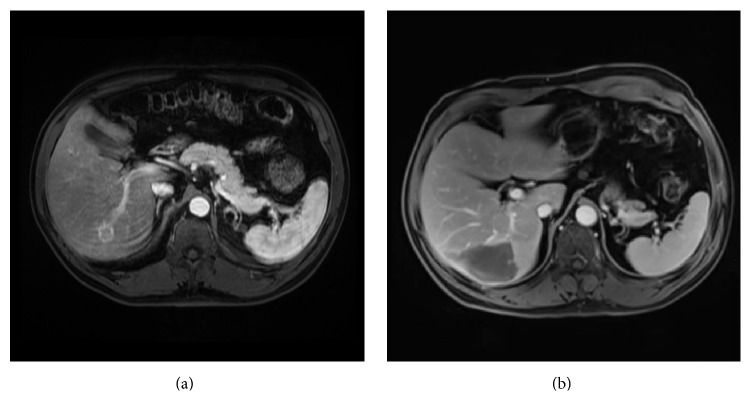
Abdominal MRI before microwave ablation shows a rounded lesion in liver segment 6, measuring 2.1 × 2.1 cm, which demonstrates T2 hyperintensity with heterogeneous internal enhancement or restricted diffusion on MRI (a). 2 months after microwave ablation of the liver, there is evidence of postoperative and postmicrowave changes in the liver, with no suspicious enhancement in the ablation bed to suggest definite residual or recurrent tumor, and no new focal liver lesions (b).
